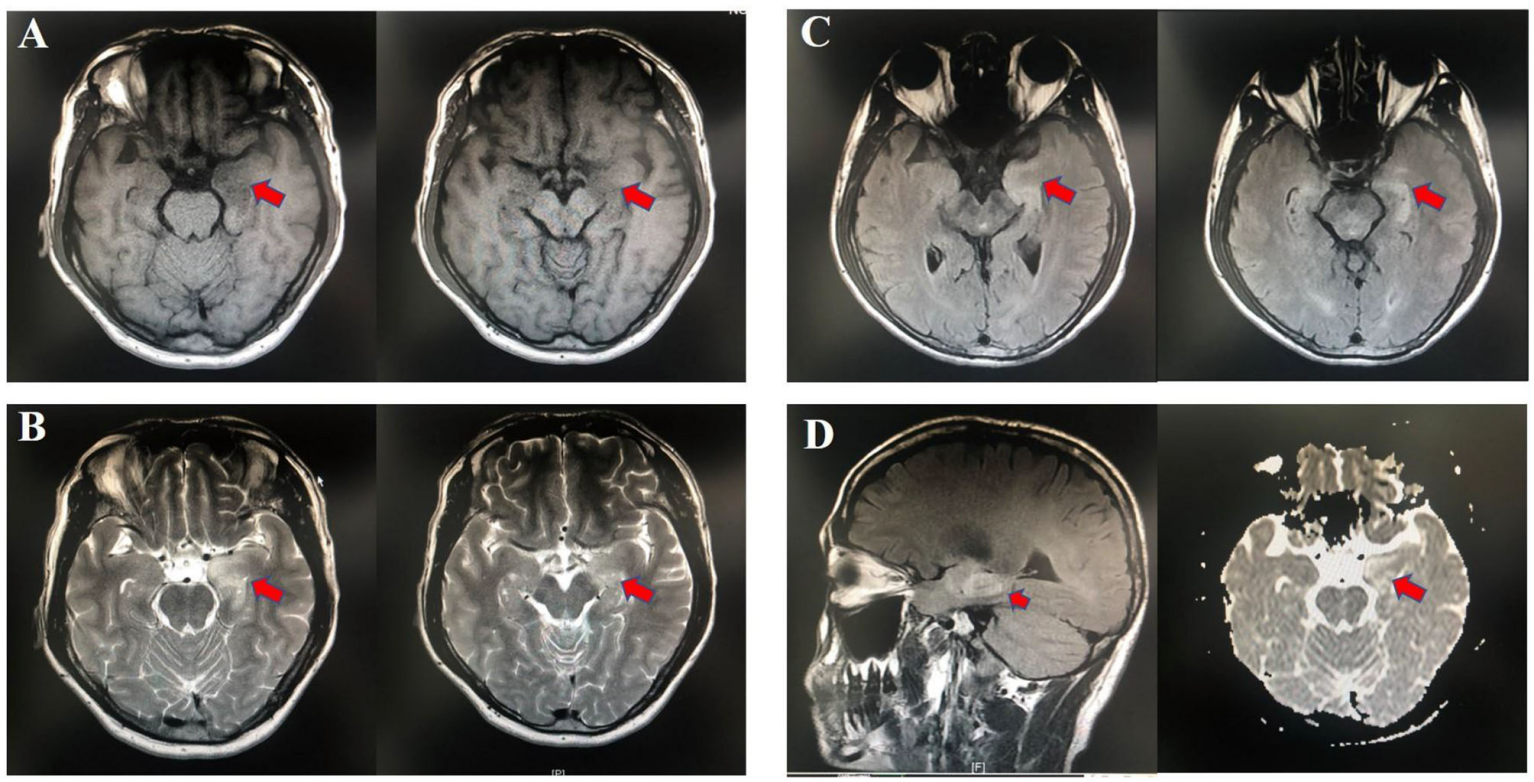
Abnormal MRI signaling (Red arrows) in the left hippocampus and parahippocampal gyrus of one patient with anti-LGI1 encephalitis. (A) TI-weighted MRI. (B) T2-weighted MRI. (C) Aixal FLAIR sequences. (D) Sagittal FLAIR MRI and axial DWI. MRI, magnetic resonance imaging; FLAIR, fluid attenuated inversion recovery; DWI, diffusion-weighted imaging.
